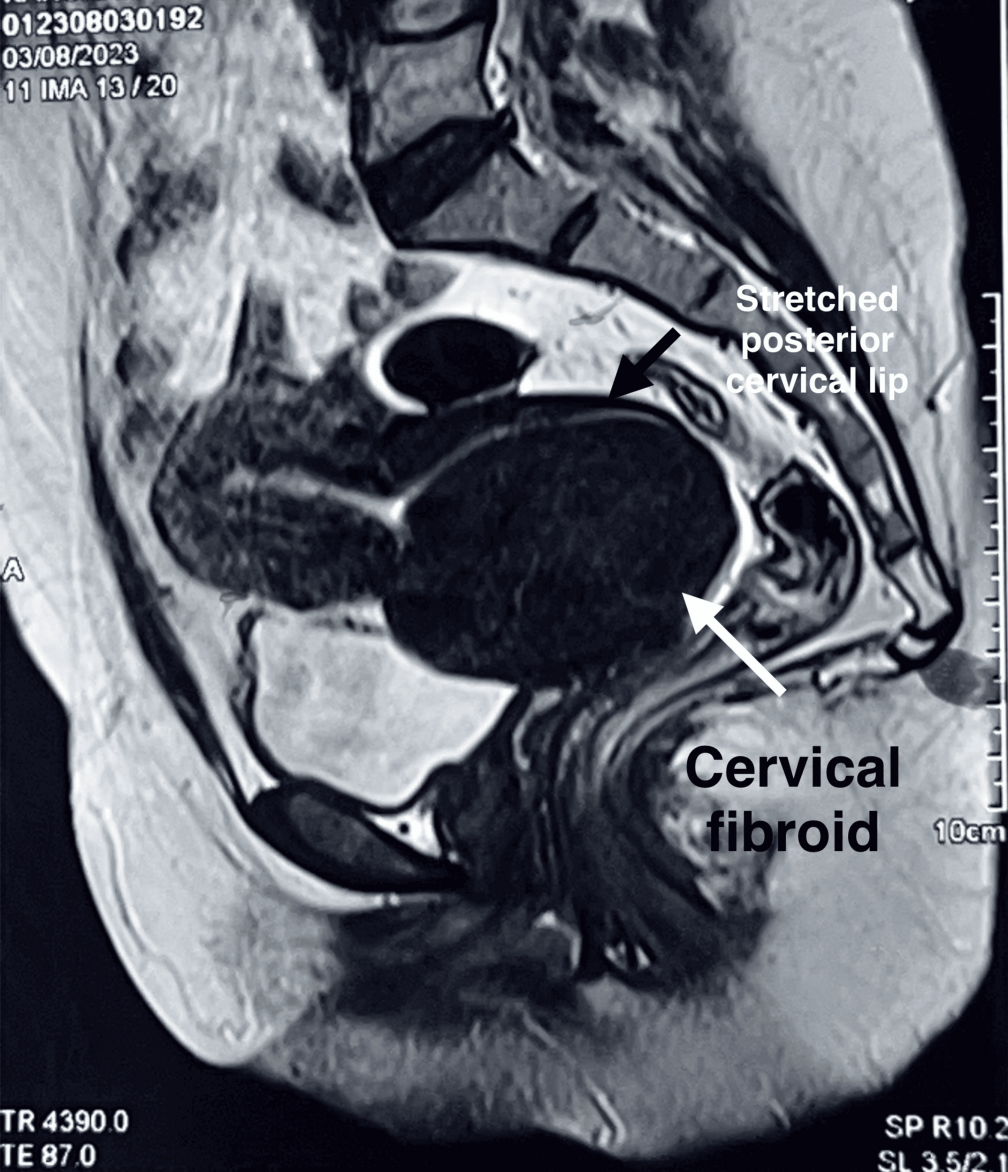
MRI was suggestive of a fibroid measuring 52 x 65 x 68 mm, involving the anterior lip of the cervix, with the posterior lip spread over it and extending into the vagina
